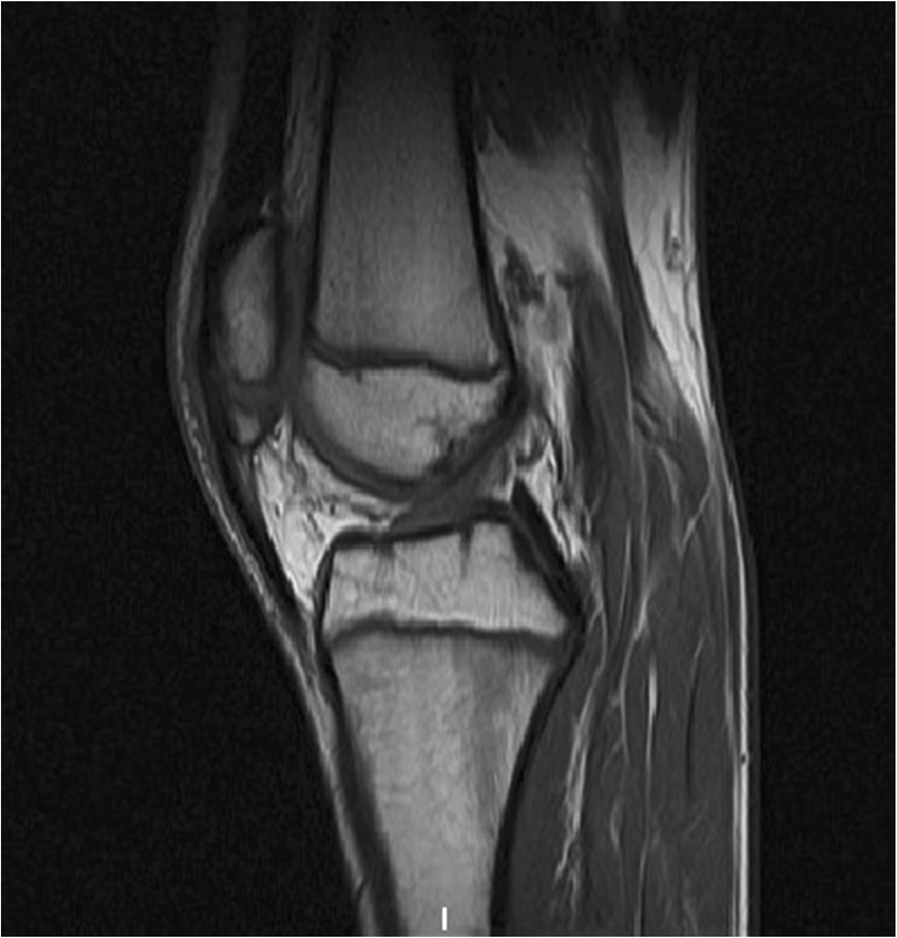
T1-weighted sagittal MRI of Case 1 at 2 weeks.
